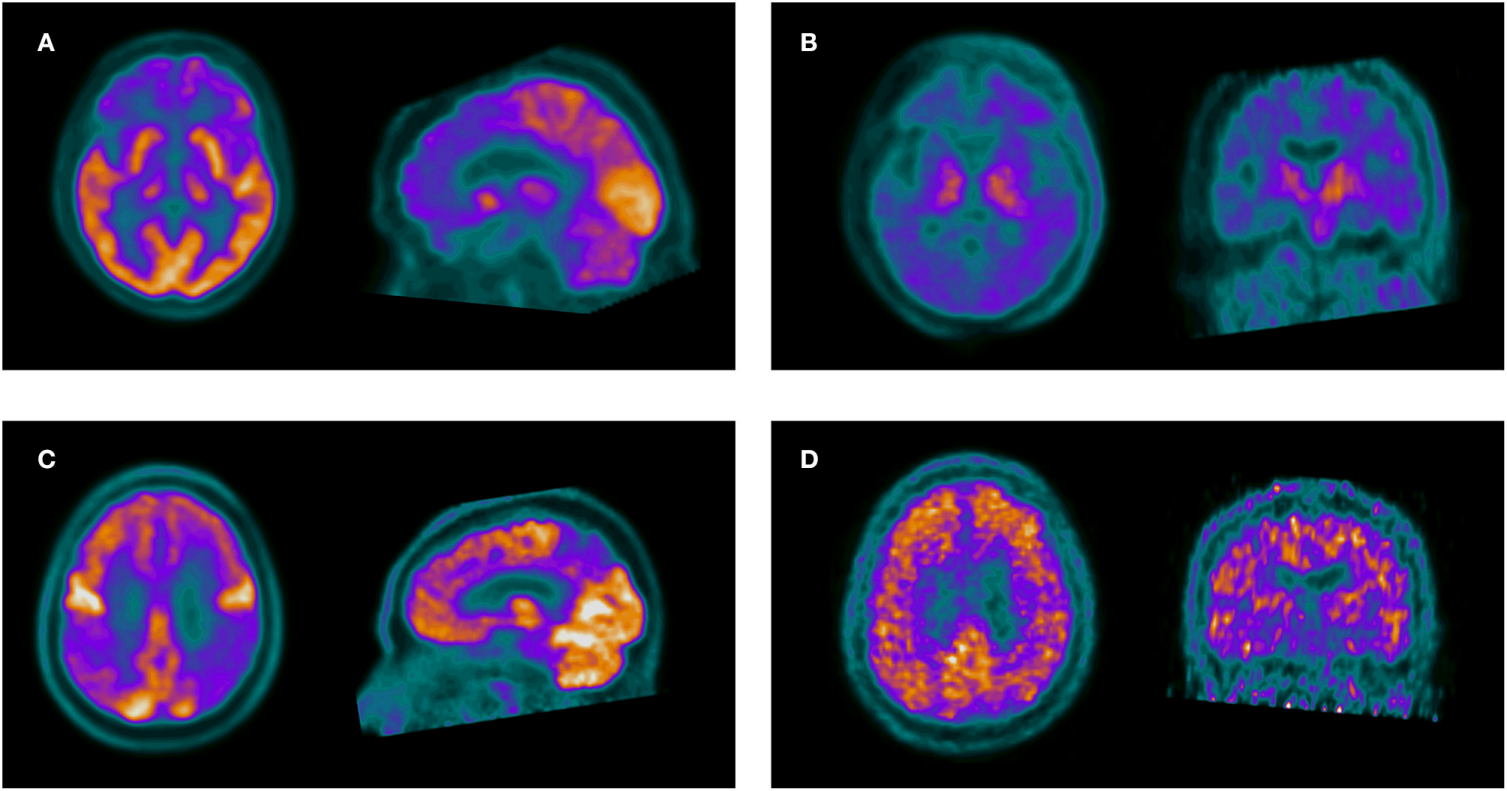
The upper row shows a 56-year-old woman with memory complaints and change in behavior, referred with overlapping symptoms of AD, FTD and a baseline diagnosis of AD. Axial and sagittal slices of 18F-FDG PET (A) show frontal hypometabolism suggestive of FTD. Axial and coronal ^11^C-PIB PET slices (B) are negative for amyloid deposition, confirming FTD. The lower row shows a 68-year-old male with symptoms of a non-fluent progressive aphasia. ^18^F-FDG PET (C) shows posterior parietal and precuneus hypometabolism suggestive of AD. ^11^C-PIB PET (D) confirms AD pathology.
